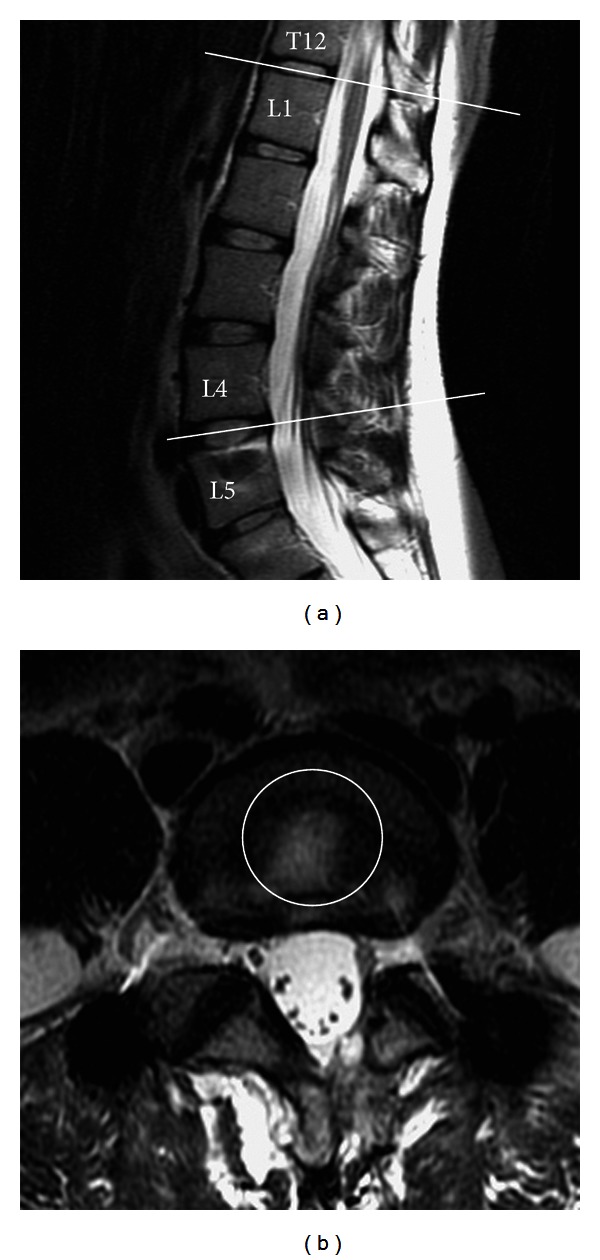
MRI T2-weighted image of a 34-year-old female. (a) Sagittal view. (b) Axial view. The axial view of each disc was retrieved in correspondence with the center of each disc in sagittal view. A circle of one centimeter in radius, region of interest (ROI), was drawn in the geometrical center of each disc. The mean signal intensity of ROI was then measured and recorded. The mean signal intensity of ROI of each level (e.g., L4-5) divided with reference level (i.e., T12-L1) was called CDS.
